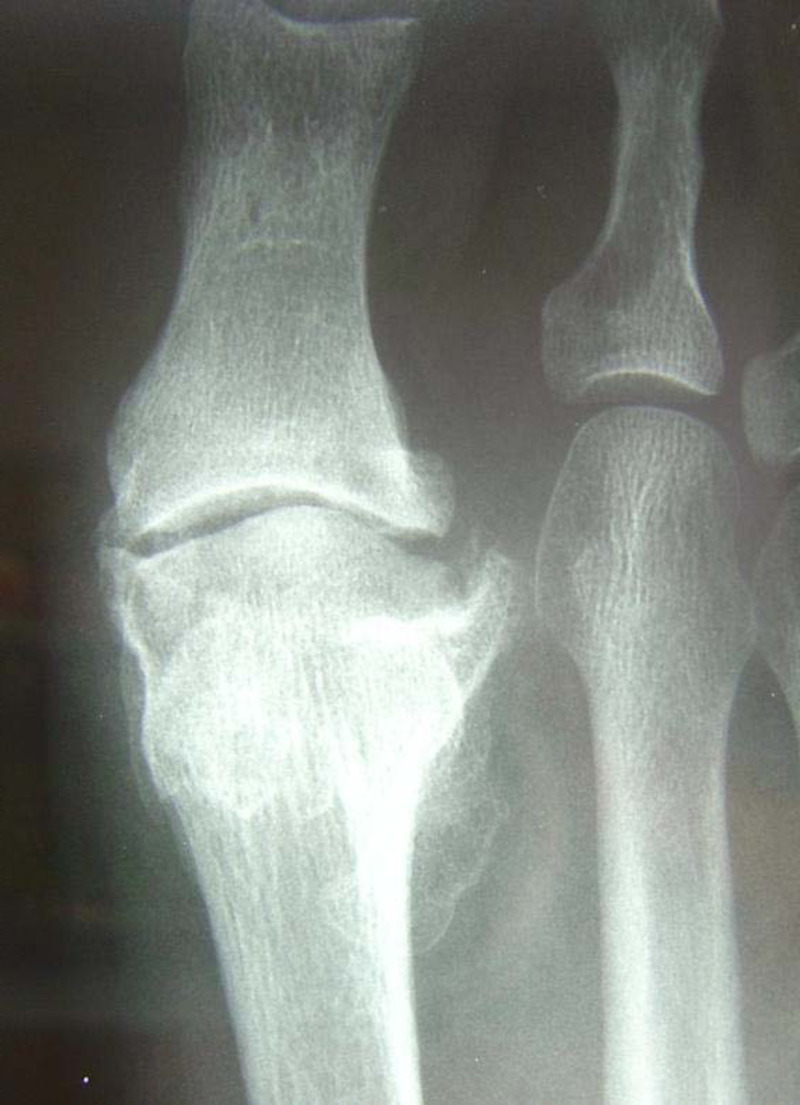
Preoperative plain film radiographs showing degenerative change
